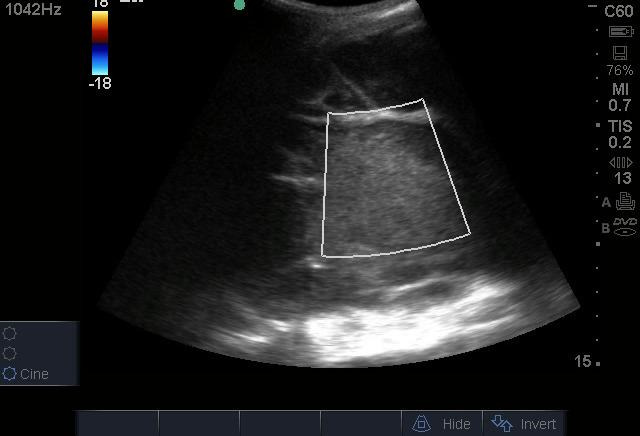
No evidence of color Doppler flow during the left flank assessment.
